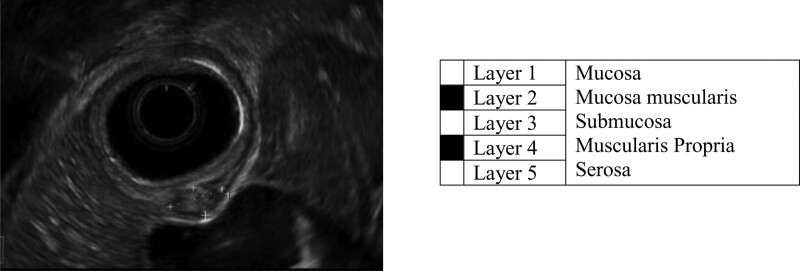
EUS showing the alternating light/dark pattern seen between the bowel wall layers (antrum). Small lesion is a submucosal mass. EUS = endoscopic ultrasound.
